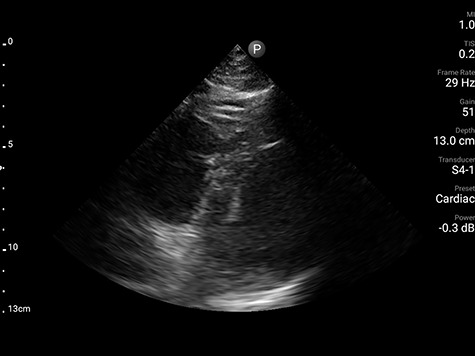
Parasternal short-axis view demonstrating dilated right ventricle (star) with flattened septum (arrow).
